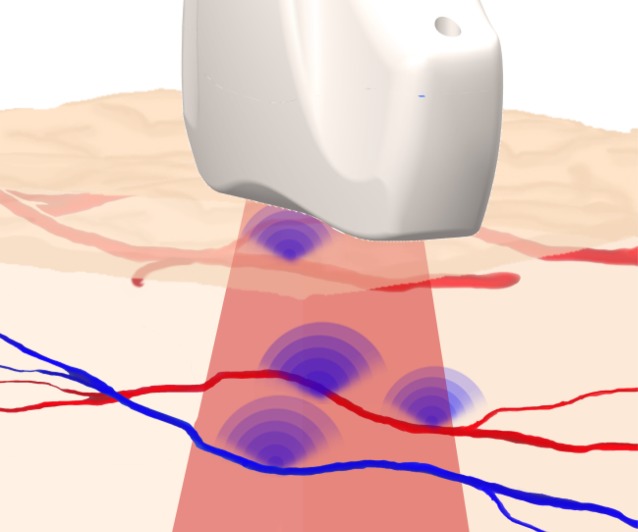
The principle of multispectral optoacoustic tomography (MSOT): tissue is illuminated with nanosecond laser pulses. Absorption of light energy by absorbers in the tissue causes a slight rise in temperature and thermoelastic expansion. The ultrasound waves thus generated are registered with a hand-held detector (courtesy of iThera Medical GmbH)
